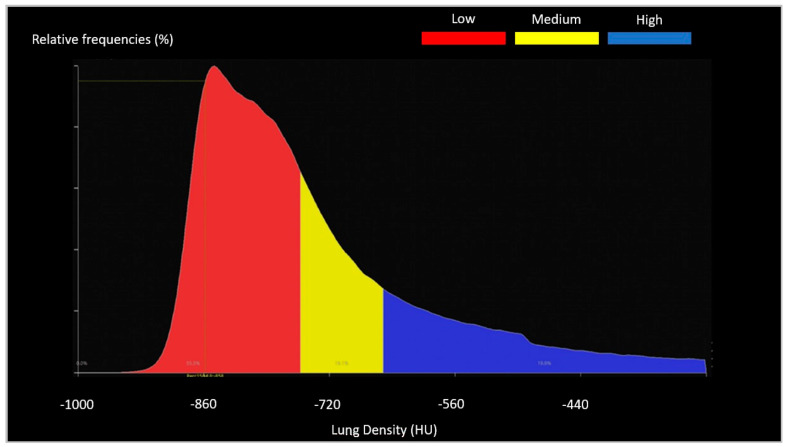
CT Lung Density Analysis: histogram of attenuation areas. The red area represents the low attenuation area [−990; −750 HU]; the yellow one represents the medium attenuation area [−750; 660 HU]; and the blue area shows the high attenuation area [−660; 0 HU].
